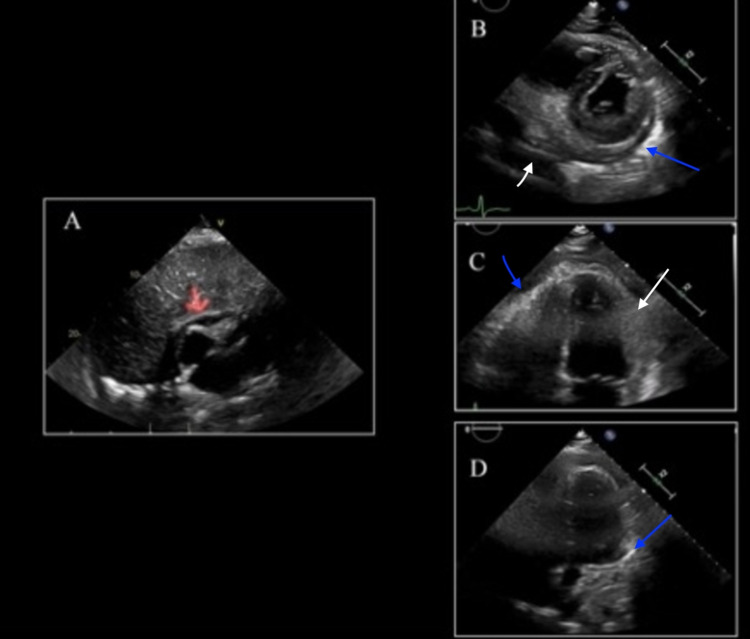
Transthoracic Echocardiogram demonstrating signs of pericarditis. (A) Subcostal view of prior TTE one month ago demonstrating small pericardial effusion with mild echogenicity of the visceral pericardium (demonstrated by red arrow). (B) Short axis view of current TTE demonstrating persistent pericardial effusion (demonstrated by white arrow) with enhanced echogenicity of the visceral pericardium (demonstrated by the blue arrow). (C, D) Four-chamber view of current TTE further demonstrating circumferential thickening (demonstrated by white arrow) and echogenicity of the visceral pericardium (demonstrated by the blue arrow).
